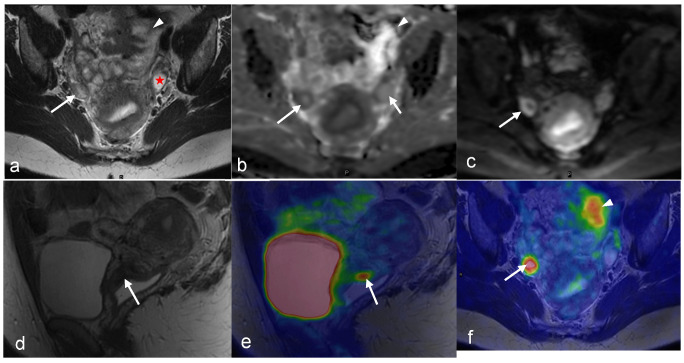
A 43-year-old woman with stage IB1 cervical cancer found to have a corpus luteum cyst in her right ovary. The cyst is visualized as a peripherally low-intensity structure with central high intensity (arrow in (b)) on ADC map and a peripherally hyperintense structure with low central intensity (arrow in (c)) on the axial diffusion-weighted image. The corresponding FDG uptake (arrow in (f)) on the fused PET/MRI is determined to be benign. In addition, the benign ovarian cyst in the left ovary is seen (star in (a)) with no pathological FDG uptake. The arrowhead in (a,b,f) shows part of the left superior corner of the bladder with corresponding FDG uptake in the urine. Sagittal T2-weighted MRI (d) and sagittal-fused PET/MRI (e) show the cervical mass (arrow in (d,e)). (Courtesy of Elisabeth Hedlund, MD, Håkan Ahlström, MD, and Björg Jónsdóttir, MD, PhD, Uppsala University, Uppsala, Sweden).
